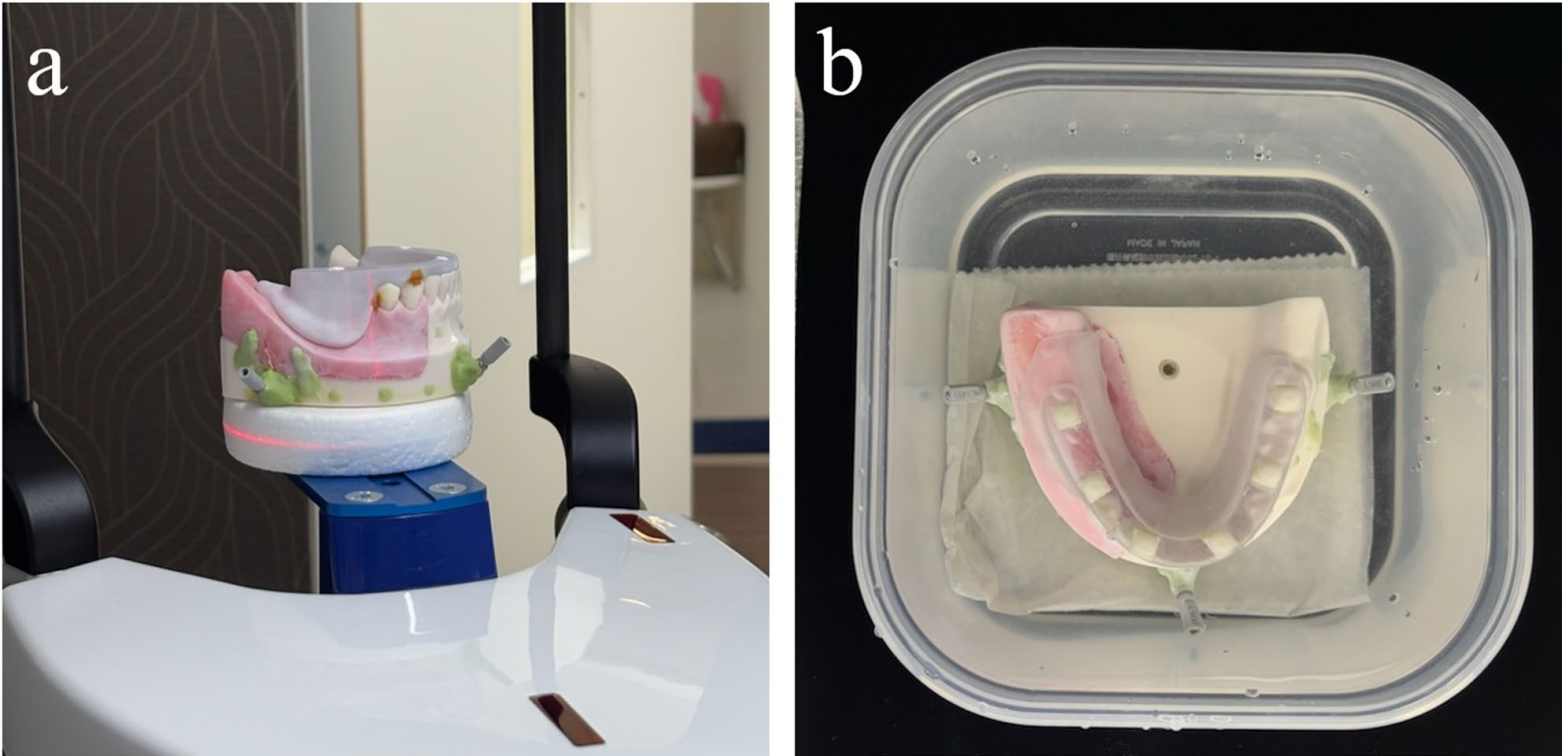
Cone-beam (CBCT) imaging conditions. a Under air conditions, the master model with CTMT was directly placed in the CBCT unit. b Under water conditions, the master model with CTMT was immersed in a container filled with water and placed in the CBCT unit
